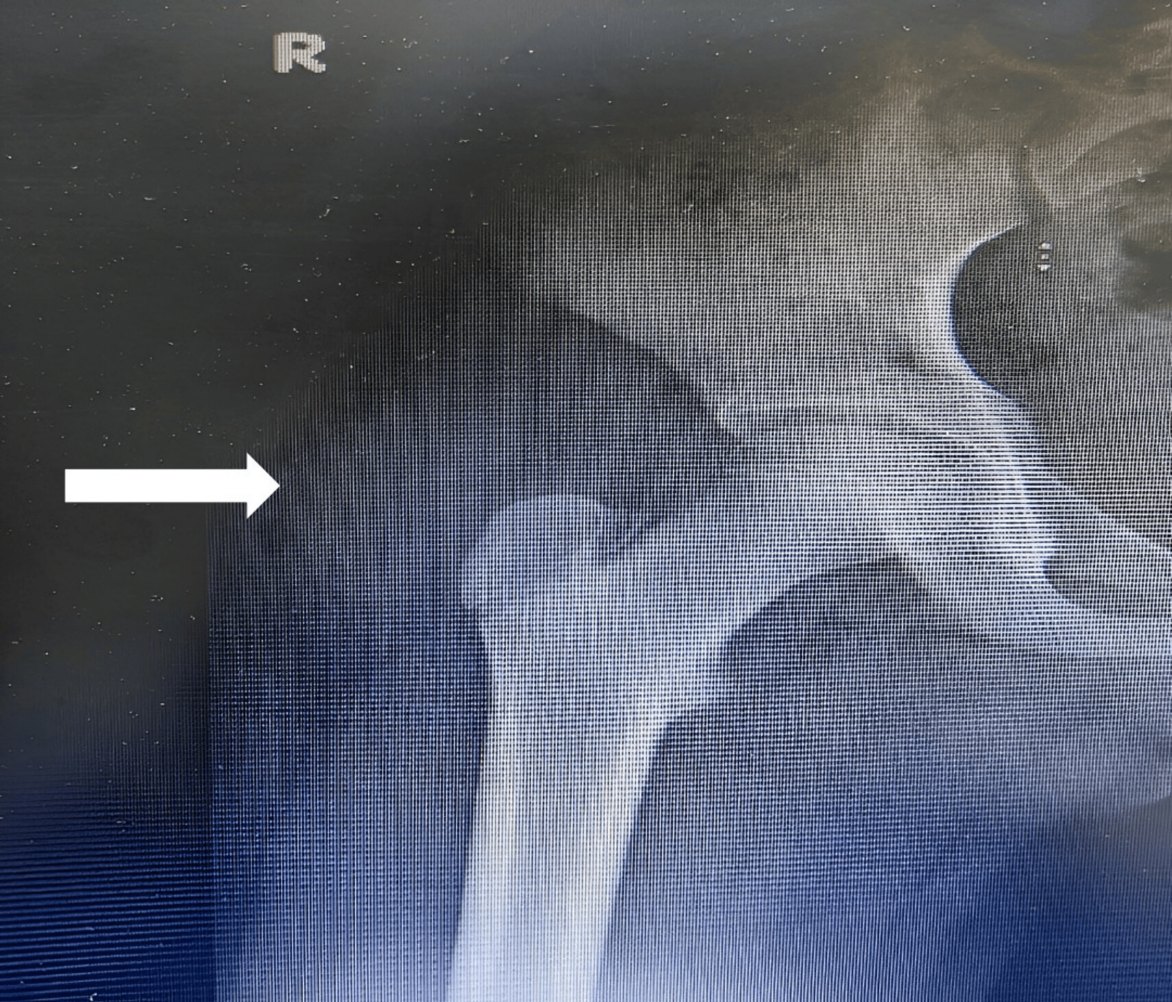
Post-resection AP X-ray right hip shows complete resection (arrow).
